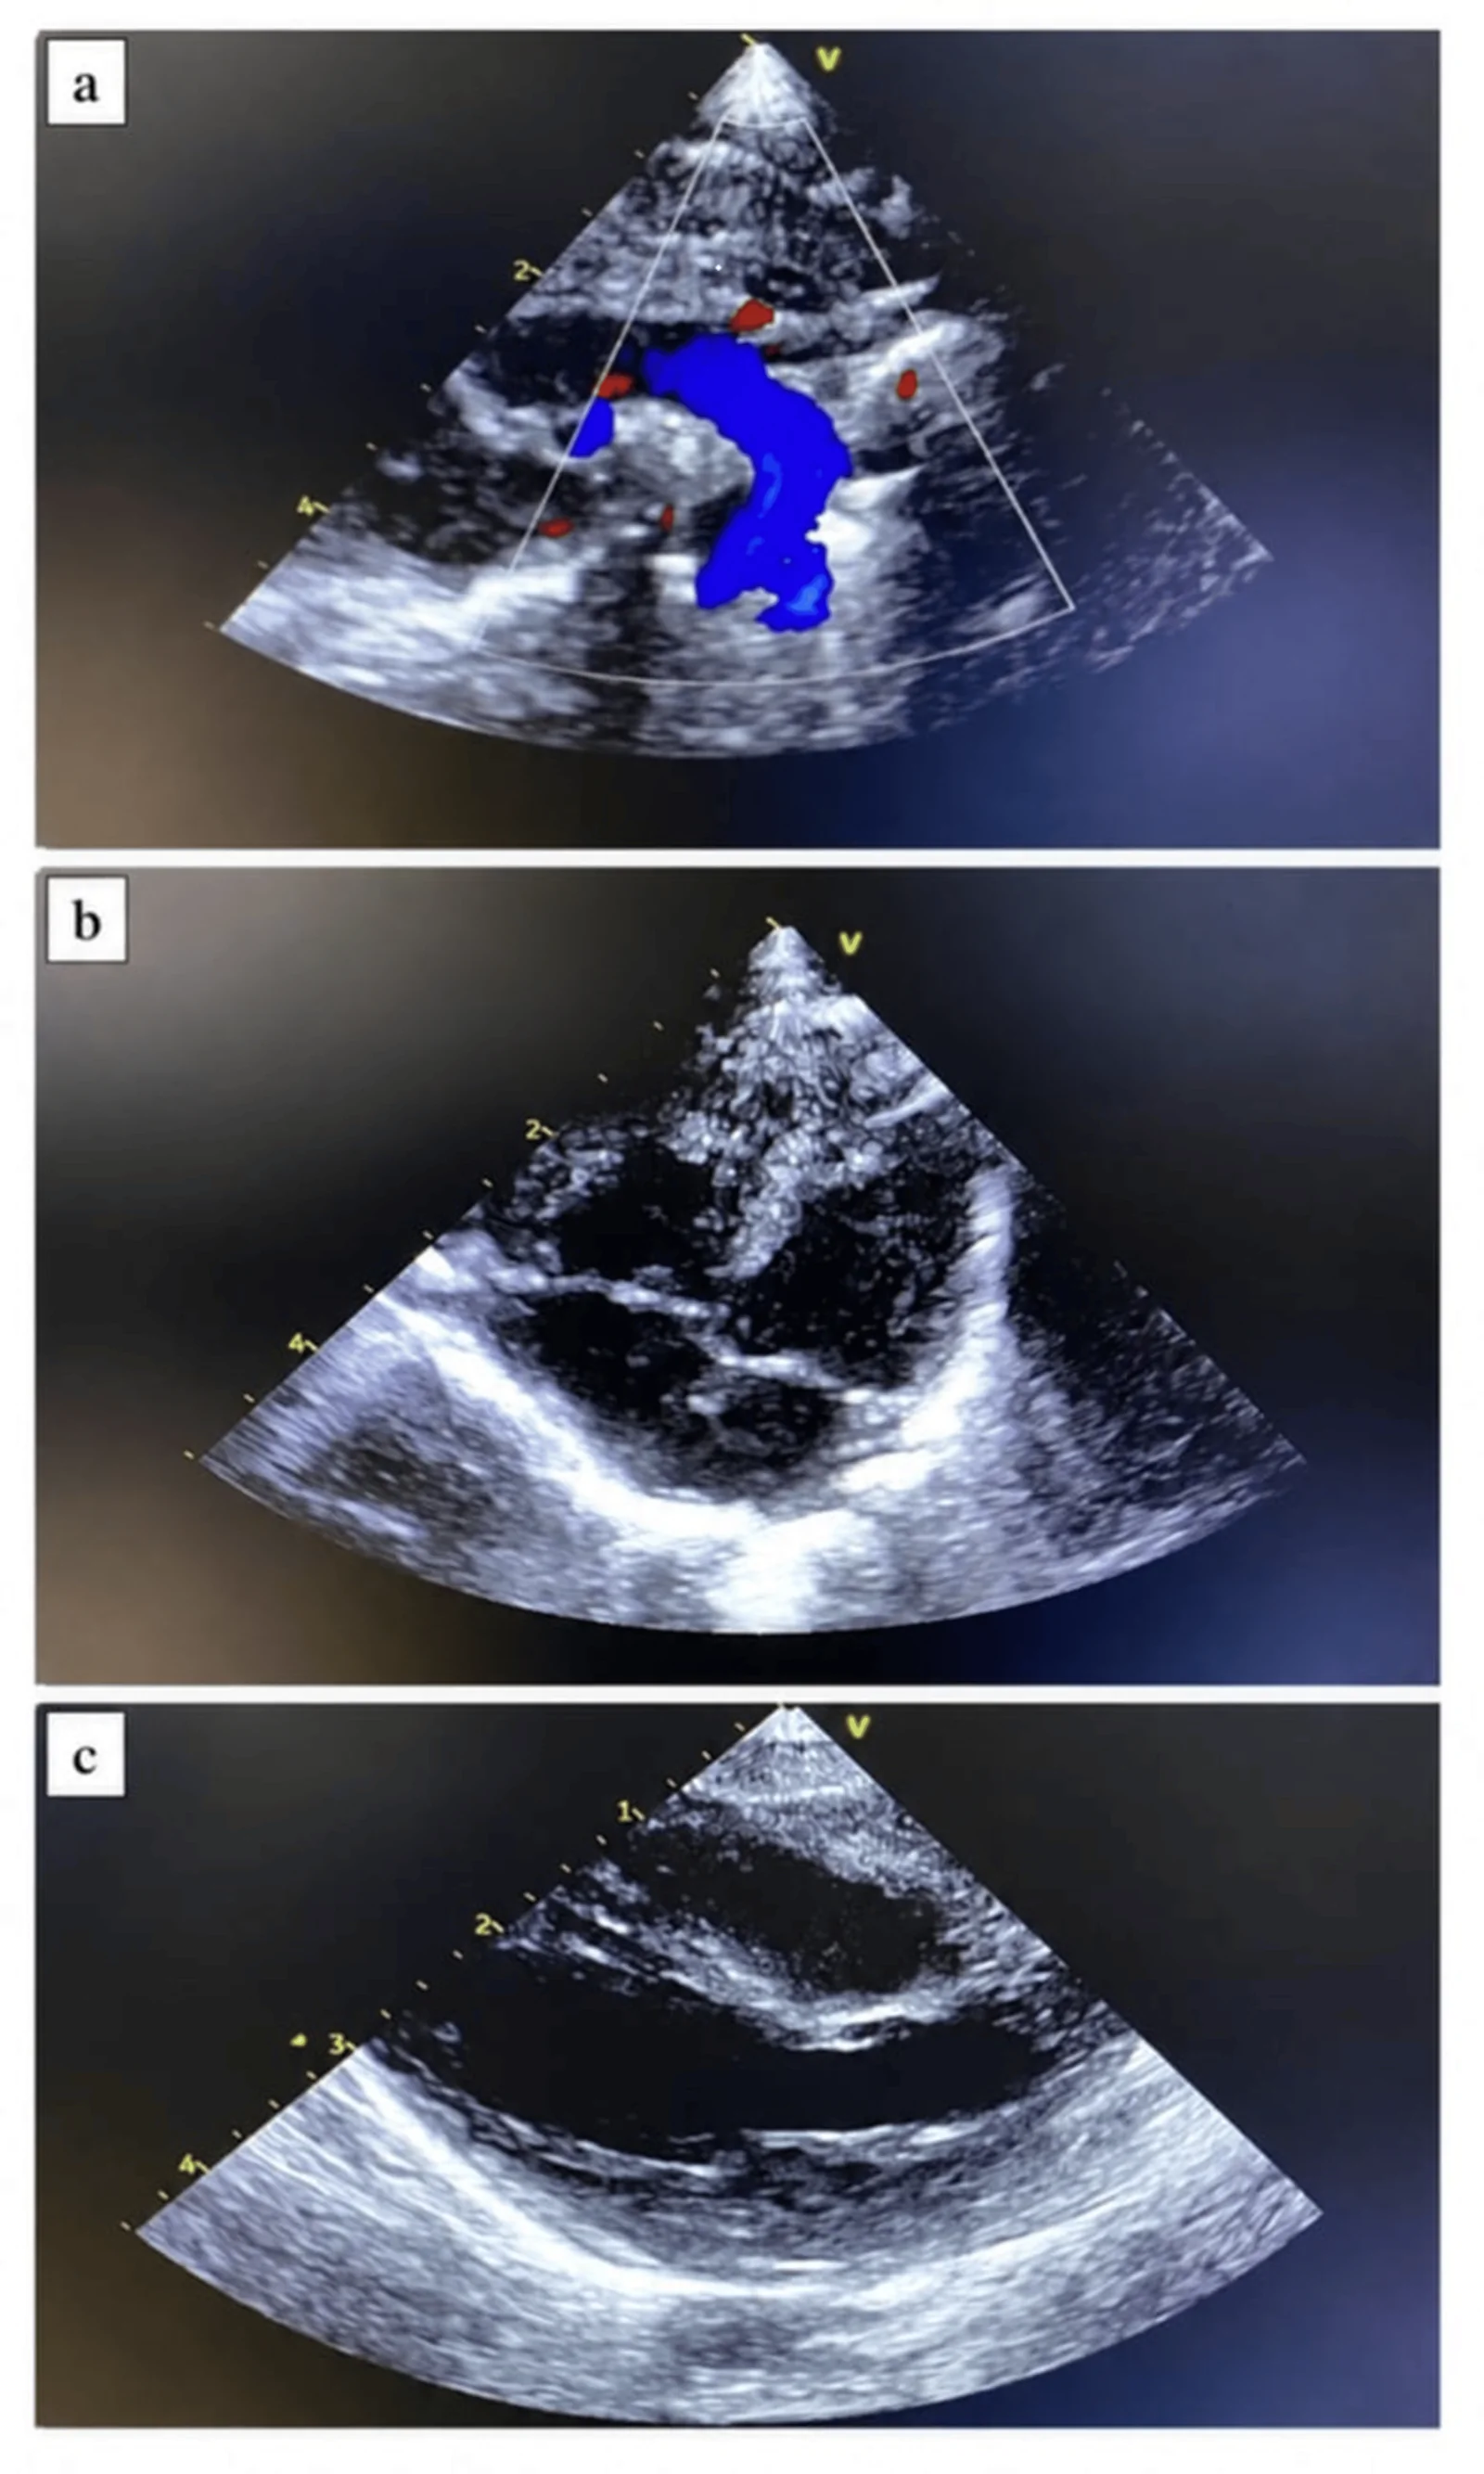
2D echocardiography report (a) Apical four-chamber view with color Doppler showing laminar flow across the atrial septum and ventricular inflow; no evidence of septal defect or valvular regurgitation. (b) Apical four-chamber view in grayscale showing normal cardiac chamber size and configuration with intact interatrial and interventricular septum. (c) Parasternal long-axis view showing normal left ventricular size and systolic function; aortic and mitral valves appear normal with no evidence of regurgitation. Overall impression: normal echocardiographic study.
